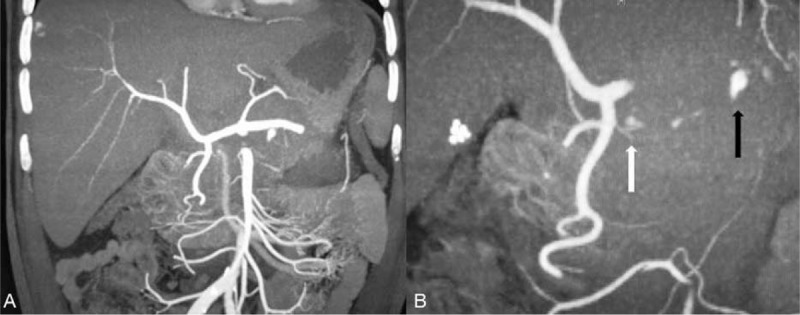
Enhanced CT scans (coronal view) before intervention. (A) The course of superior mesentery artery and celiac trunk. (B) Black arrow shows extravasation from IPDA and white arrow shows extravasation from GDA. CT = computed tomography, GDA = gastroduodenal artery, IPDA = inferior pancreaticoduodenal artery.
